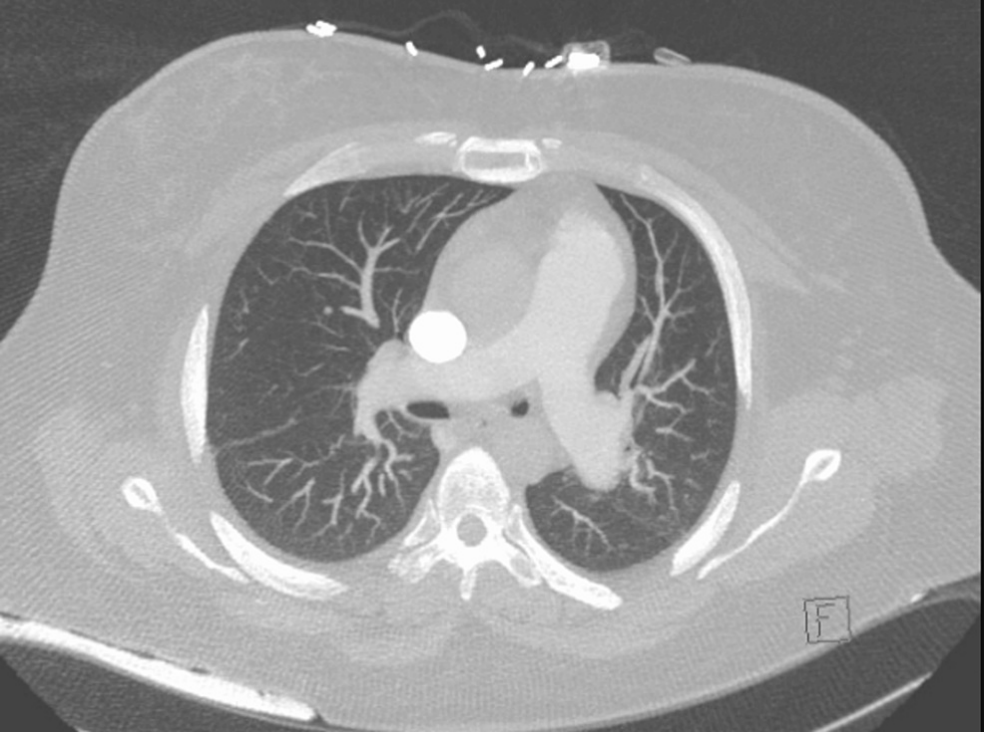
Computed Tomography Angiogram of the Chest
